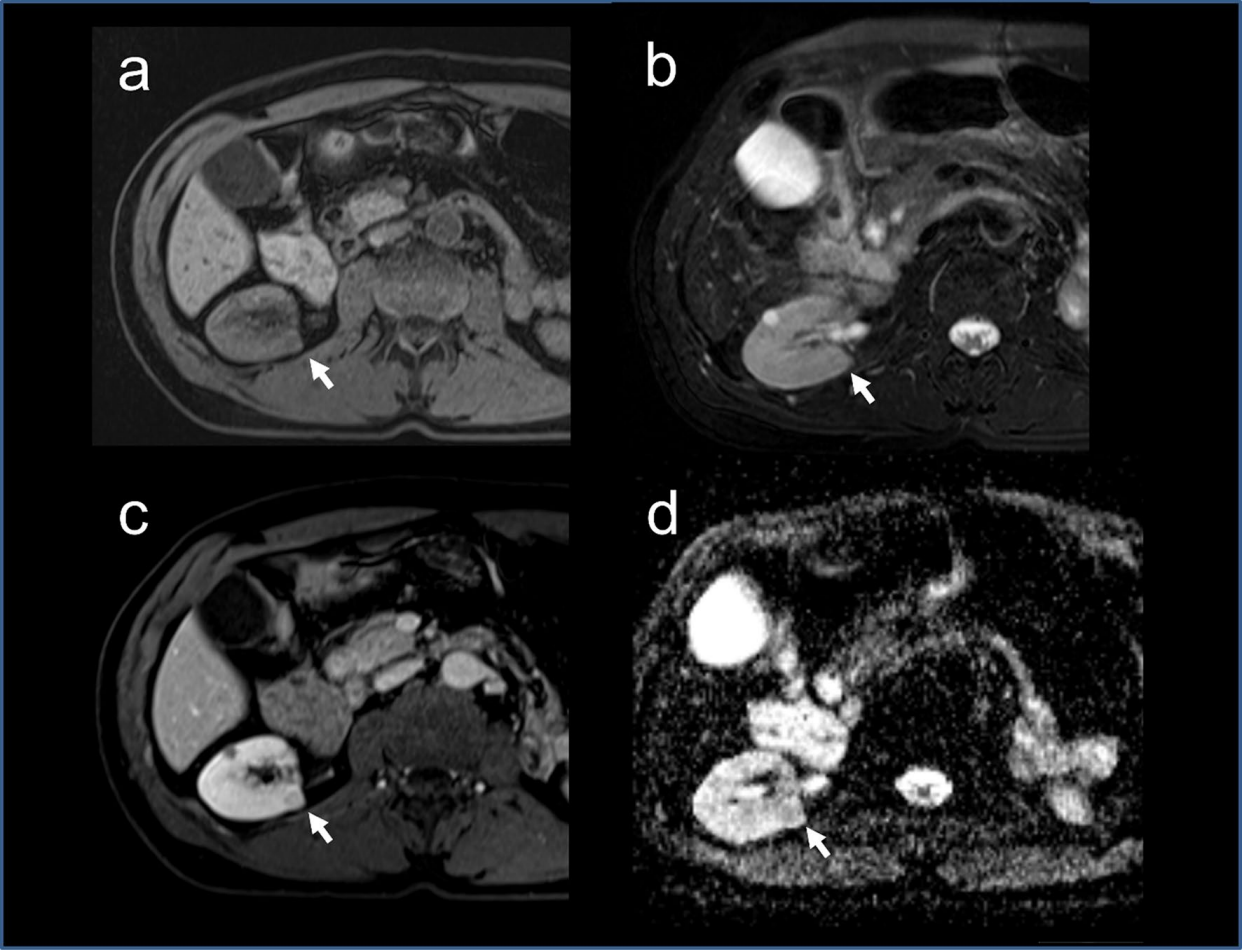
Radiological diagnosis of RCC in a GIST patient. MRI showing a coronal section of the abdomen with renal lesion (arrows). (a) T1 FS image showing a mild low signal intensity of a right posterior 8 mm renal mass. (b) T2 FS image showing increased intensity of the renal lesion. (c) T1 FS image showing hyperenhancement of the lesion after contrast injection. d. ADC MAP showing diffuse restriction of the lesion.
